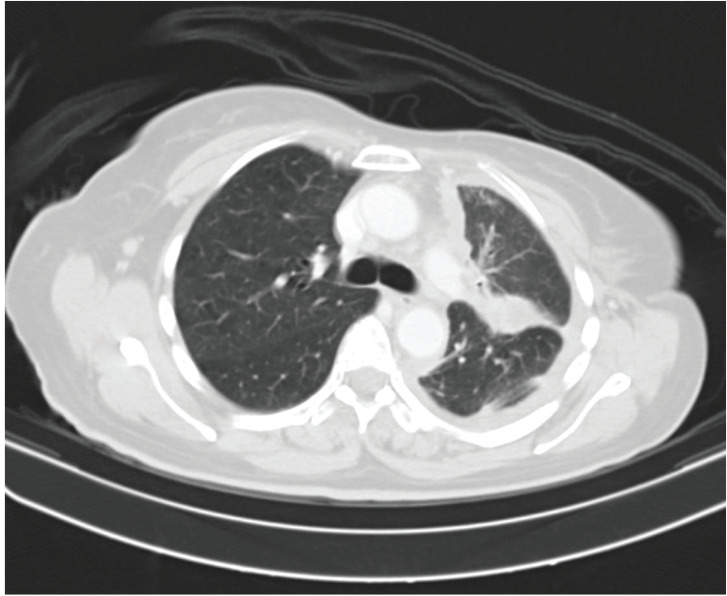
Contrast enhanced computed tomography (CECT) scan showing decrease in left sided pleural effusion with near stable diffuse pleural thickening along with decrease in consolidation.
